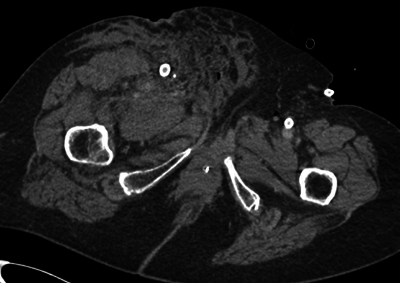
An axial CT showing extensive inguinal blood at the level of the right superficial femoral artery.
